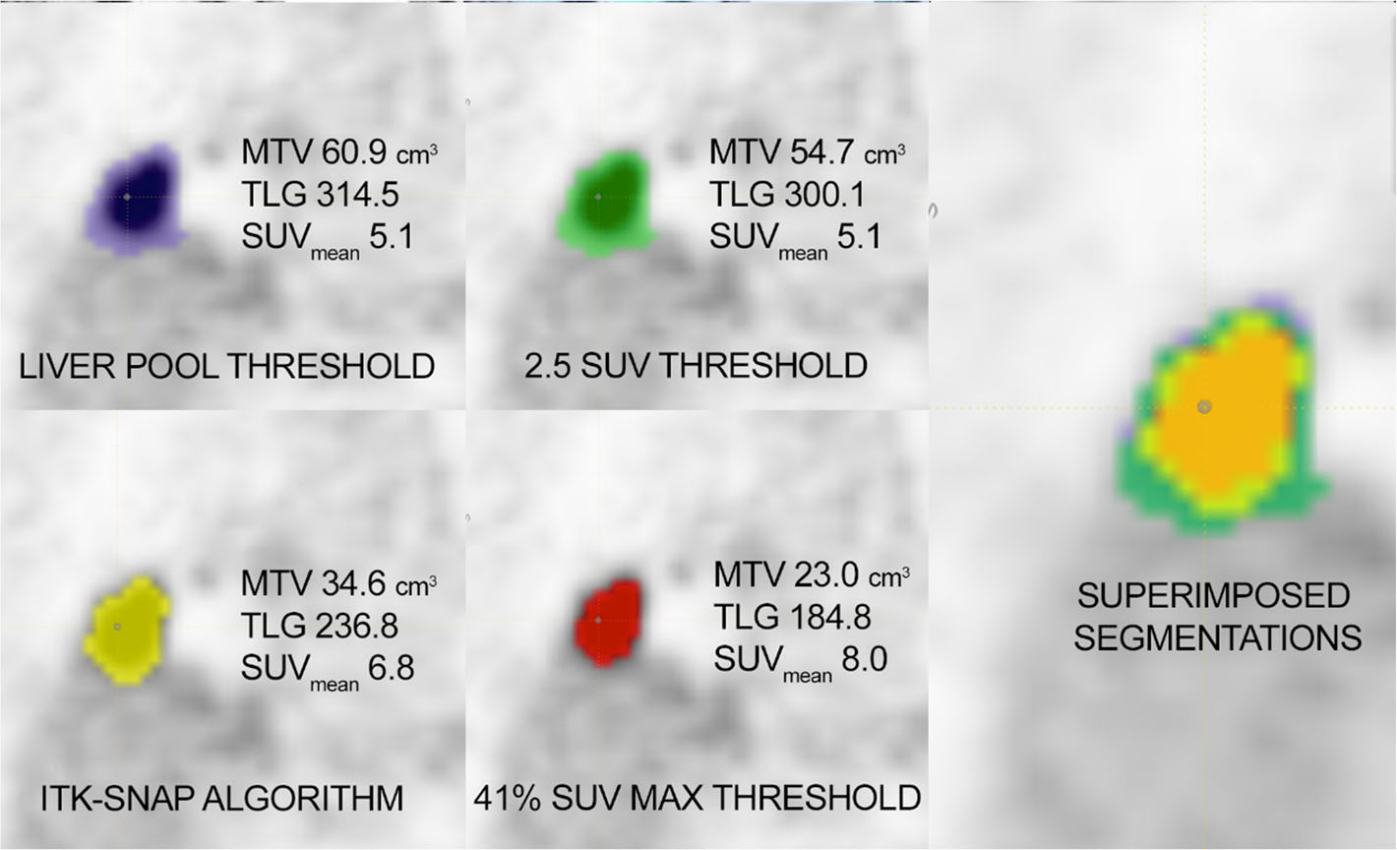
Different volumes of interest on PET images of a primary lung adenocarcinoma, produced by different segmentation techniques (presented with different colours), resulting in different values of semiquantitative metabolic parameters. *MTV* Metabolic tumour volume, *PET* Positron emission tomography, *TLG* Total lesion glycolysis, *SUV*mean Mean standardised uptake value
